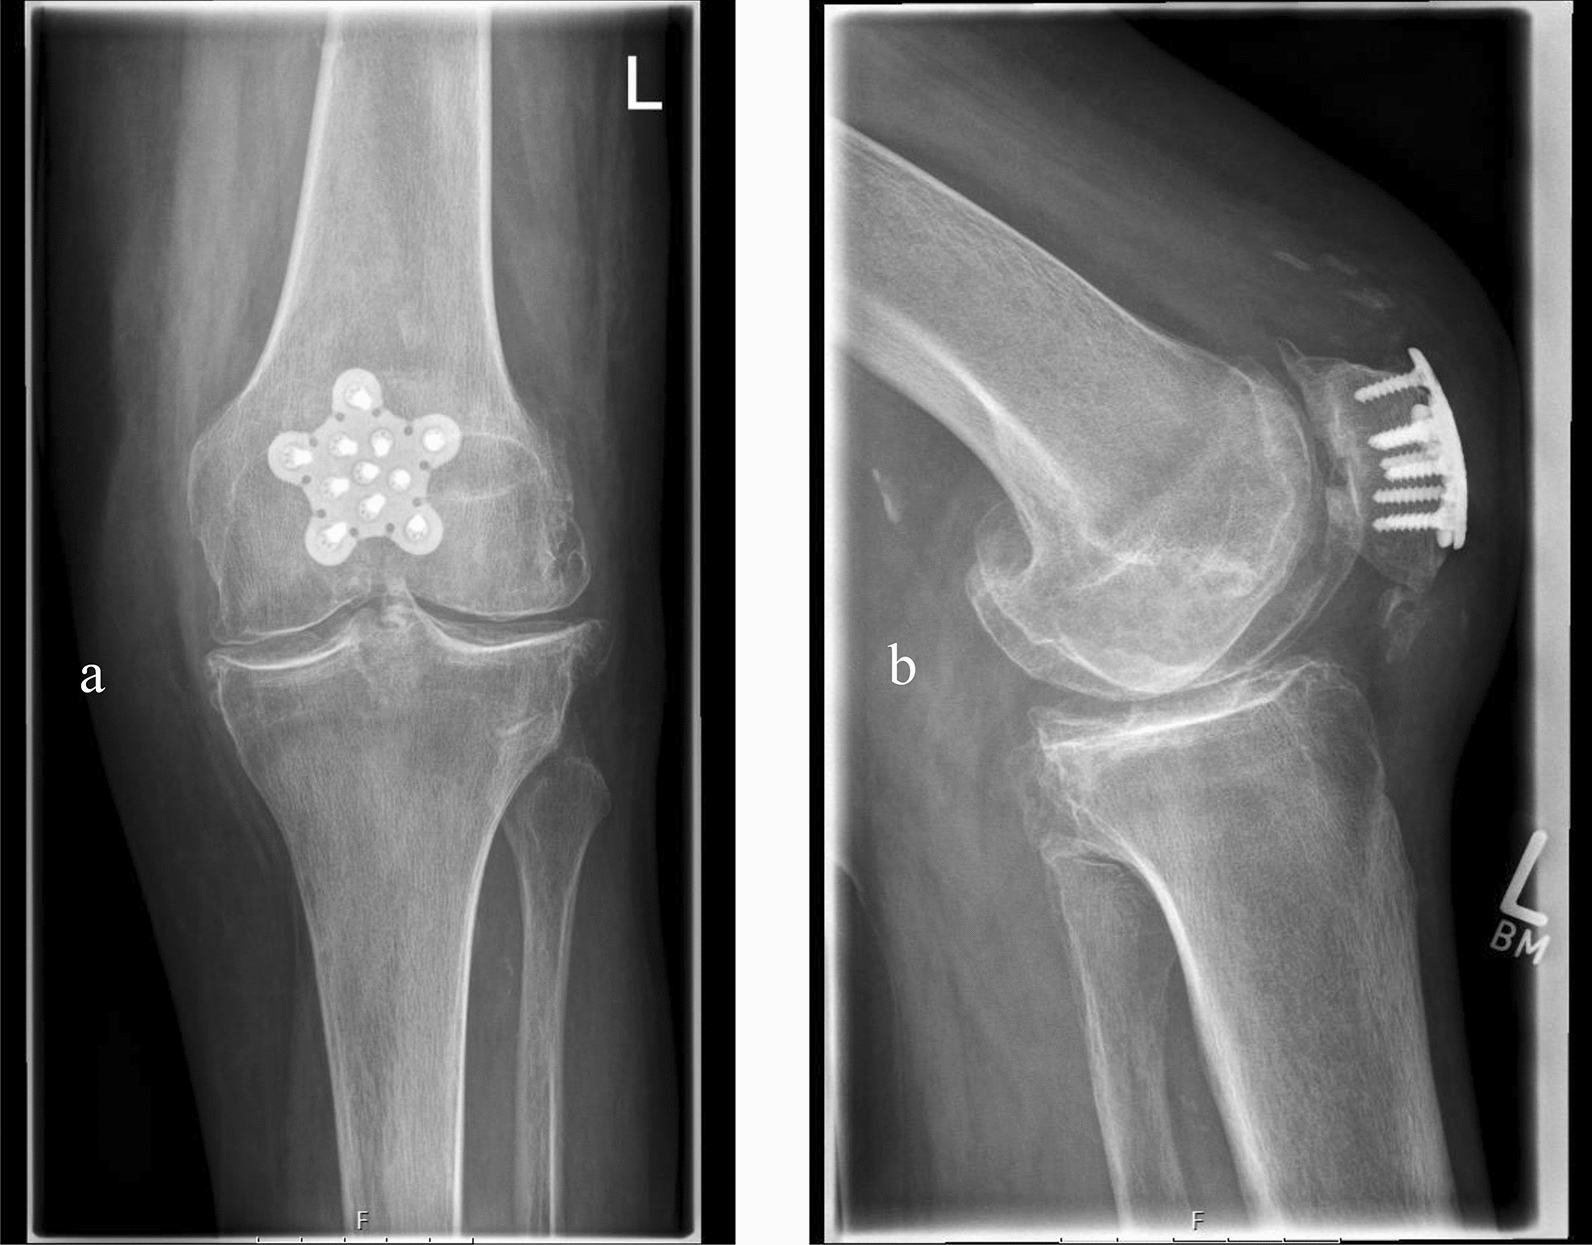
a/p (a) and lateral (b) X-ray of a multifragmentary patella fracture after operative treatment using a locking plate
